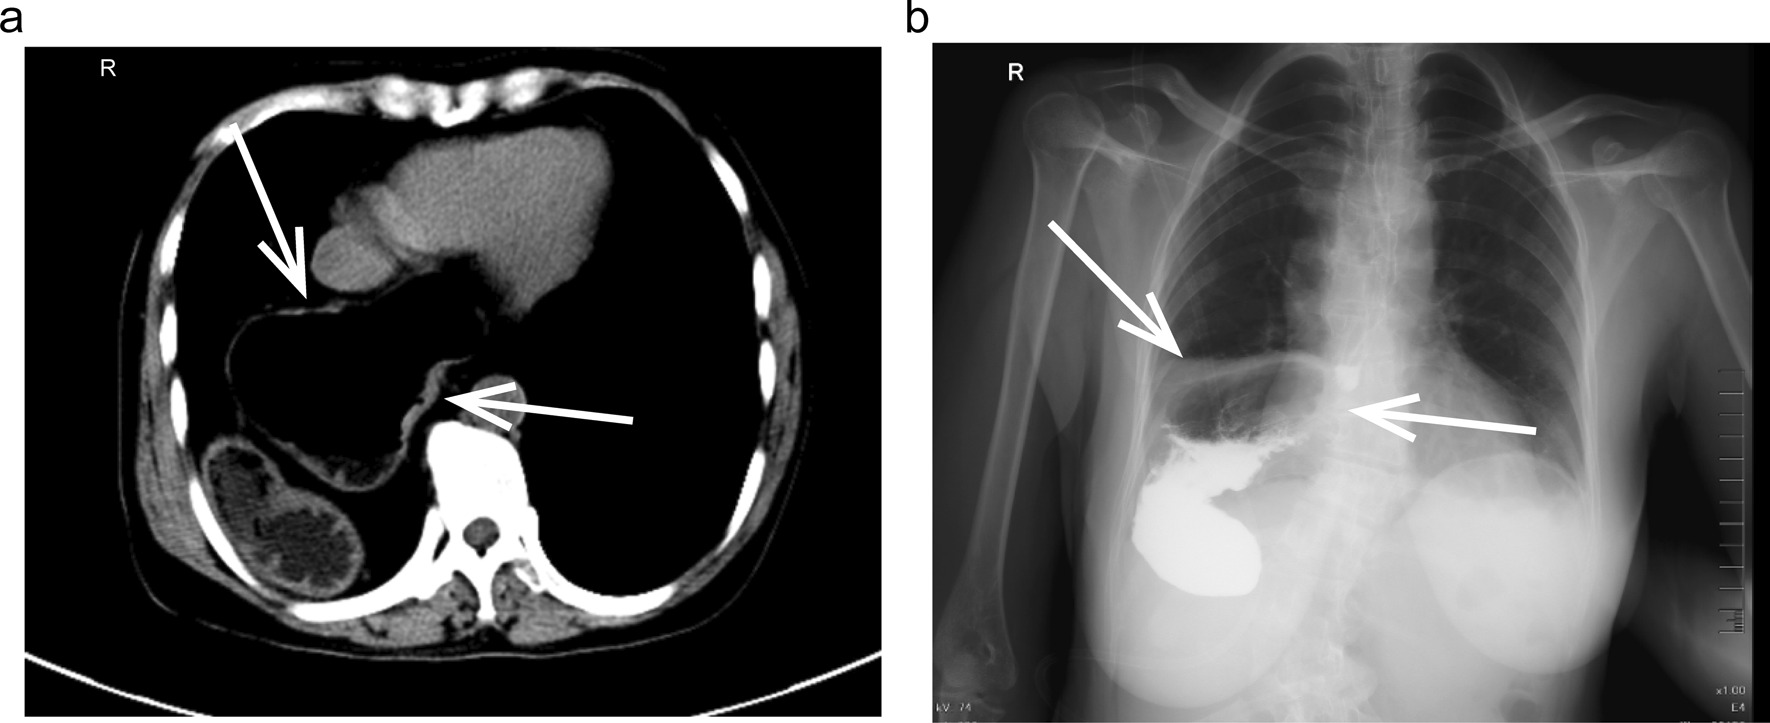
Pre-operative imaging evaluations of the patient. a CT-scan of the abdomen exhibiting the giant hiatal hernia (white arrows). b Barium swallow showing the giant hiatal hernia (black arrows)
